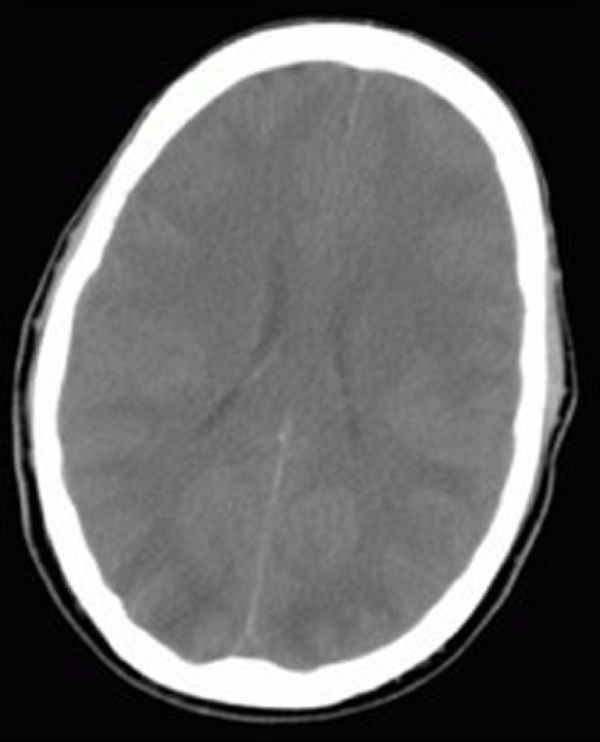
Non-contrast computed tomography showing diffuse cerebral edema.
